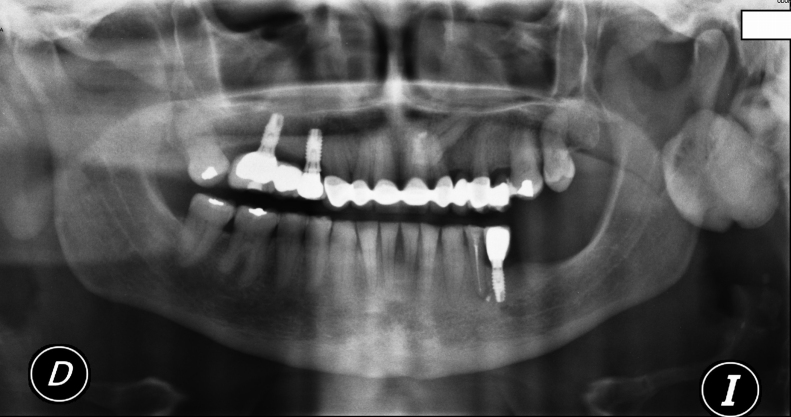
Panoramic X-ray view showing a radiopaque lesion located in the middle of the left mandibular ascending ramus.
